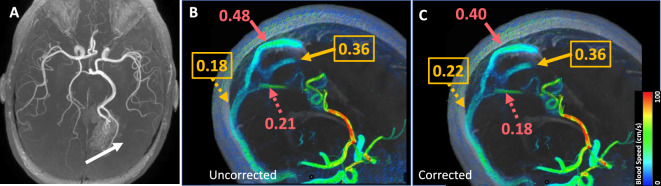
Improvement in flow visualization and flow quantification following manual background phase error correction. In a 33-year-old female, a Spetzler-Martin grade 4 AVM in the right basal ganglia (white arrow) is shown on (A) 3D TOF MRA and in oblique sagittal views with 4D Flow MRI (B and C). Low velocity arrows are seen in the soft tissues in (B), which decrease after phase error correction in (C). There is a discrepancy between venous inflow in the superior sagittal (red solid arrow) and straight (red dotted arrow) sinuses and venous outflow in the left (orange solid arrow) and right (orange dotted arrow) transverse sinuses prior to correction (0.48 + 0.21 > 0.36 + 0.18) (B), which improves after correction (0.40 + 0.18 = 0.36 + 0.22 = 0.58 L/min) (C).
